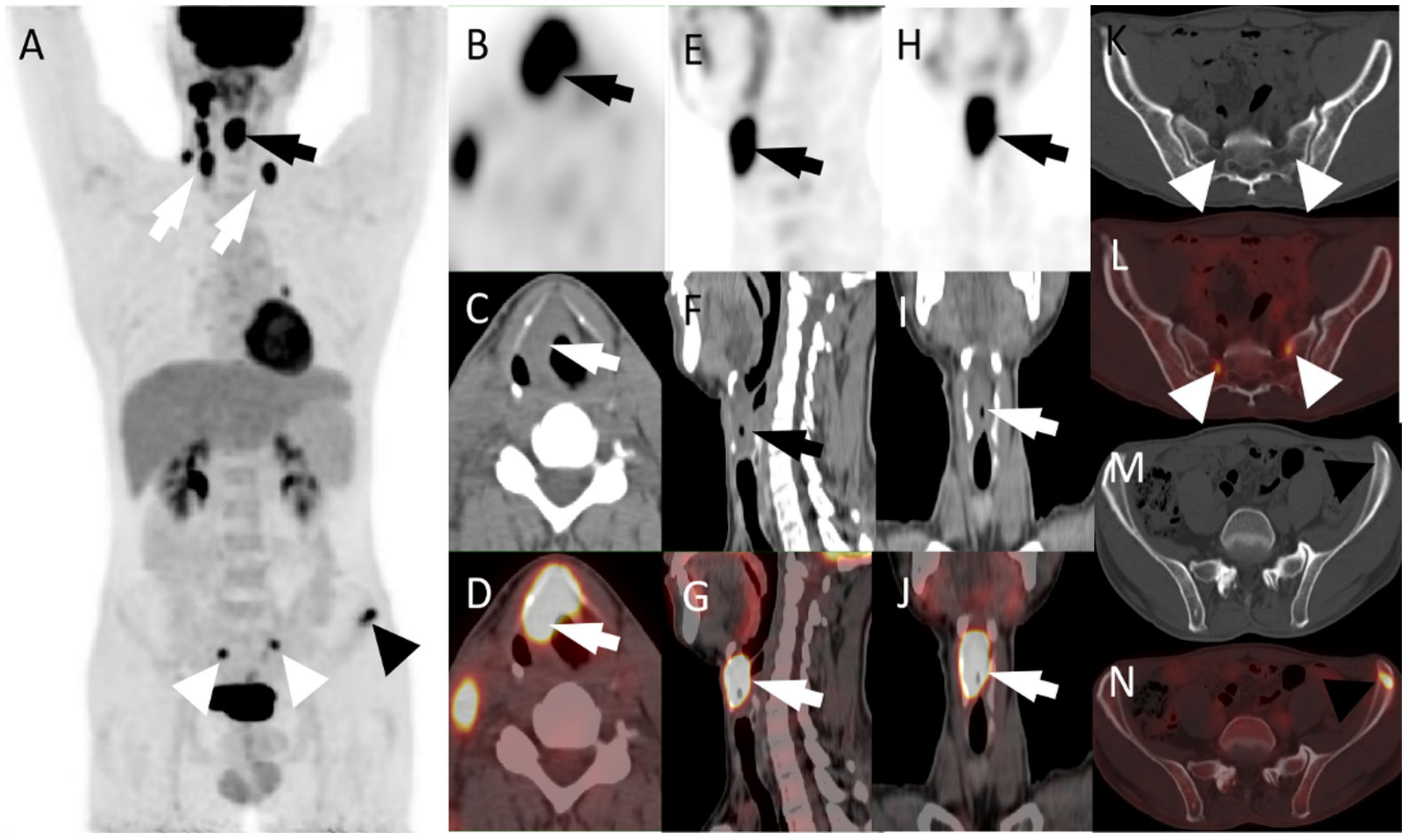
(A) The maximum intensity projection of the PET/CT scan shows increased ^18^F-FDG uptake in the right larynx (black arrow), bilateral supraclavicular area and right cervical area (white arrow), bilateral sacrum (white triangle), and left ilium (black triangle). (B, PET; C, CT; D, PET/CT fusion images) axial images of laryngeal mass. (E, PET; F, CT; G, PET/CT fusion images) sagittal images of the laryngeal mass. (H, PET; I, CT; J, PET/CT fusion images) coronal images of the laryngeal mass (SUV_max_ = 17.0; arrows). Besides, the axial CT (K,M) and fused PET/CT (L,N) images show that increased ^18^F-FDG uptake of bilateral sacrum (SUV_max_ = 8.0) and left iliac crest (SUV_max_ = 7.1) in the absence of obvious structural alterations. CT, computed tomography; ^18^F-FDG, fluoro-18-fluorodeoxyglucose; PET, positron emission tomography; SUV_max_, maximum standardized uptake value.
